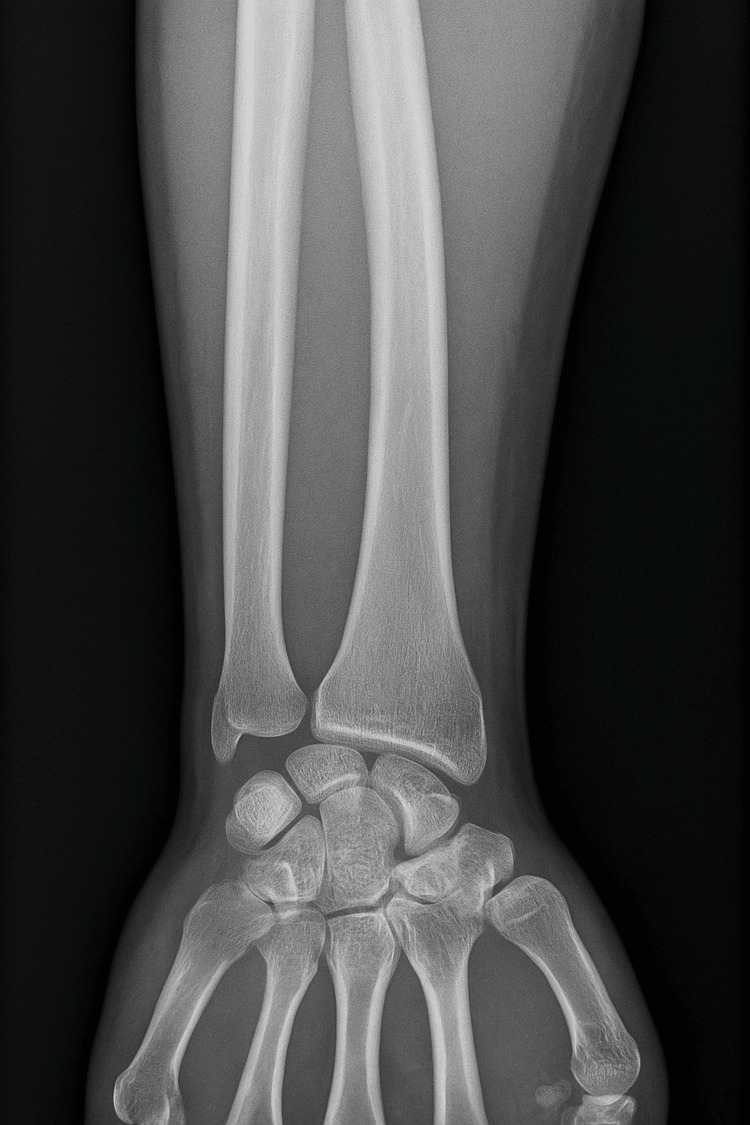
Conventional radiography of the right wrist and forearm. The posteroanterior view obtained following the trauma reveals no fractures or radio-opaque foreign bodies, confirming the absence of associated osseous injury.
